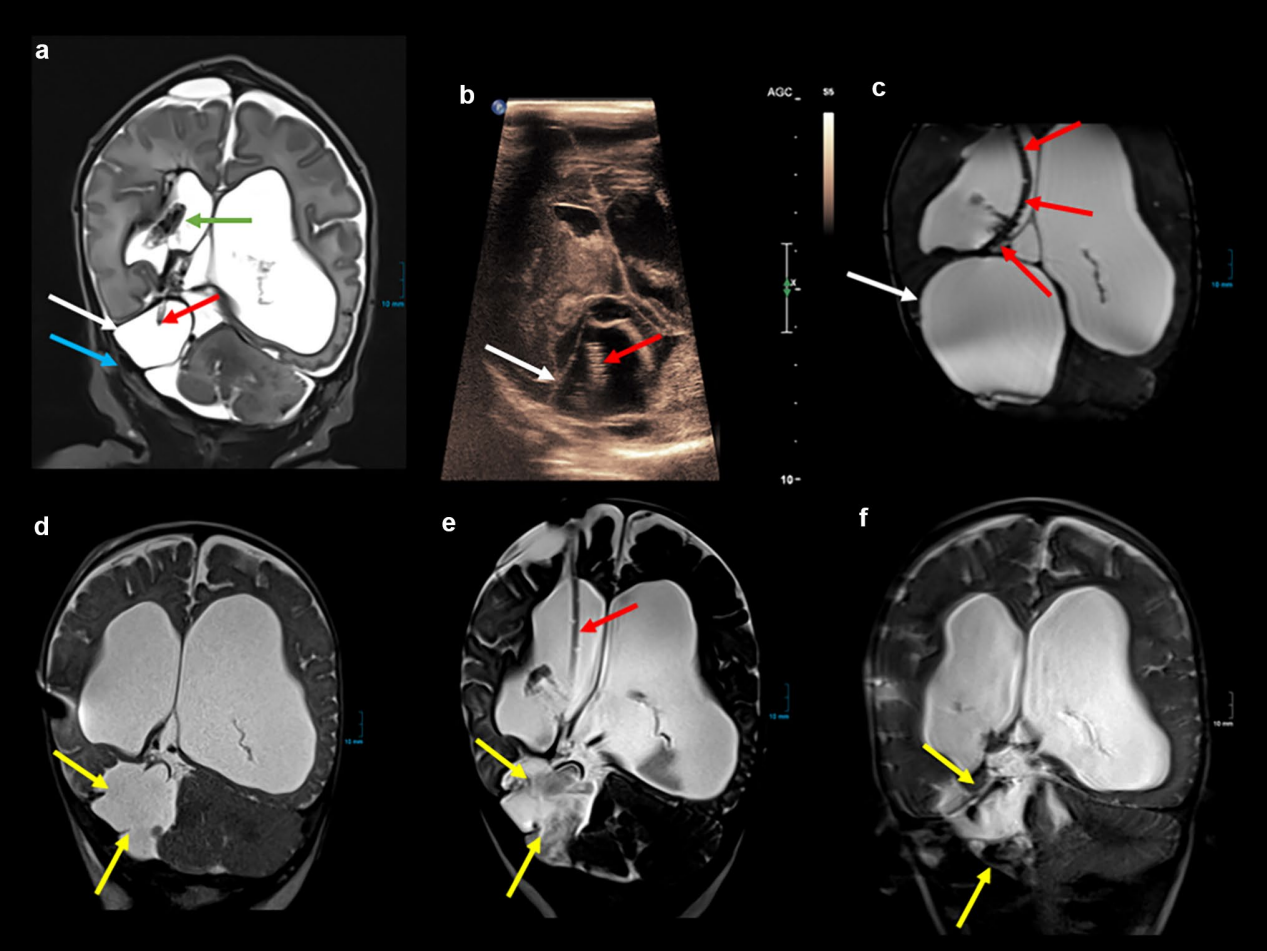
a, b MRI (a: T2 TSE) and transfontanellar ultrasound (b) both in coronal orientations after surgery: ventricular catheter (red arrow) with connection of the cyst (white arrow) with the right lateral ventricle with some bleeding into the ventricle (green arrow); the cyst has developed at the level of the tentorium next to the transverse sinus (blue arrow). c MRI (T2 CISS in coronal multiplanar reconstruction) at 3 months with recurrence of the cyst (white arrow) because the catheter slipped out of the cyst (red arrows). d MRI (T2 HASTE in coronal orientation) after supratentorial minicraniotomy with fenestration of the cyst (supra- and infratentorial, yellow arrows), consecutive reduction in cyst size. e MRI (T2 HASTE in coronal orientation): due to persistent enlargement of the supratentorial ventricular system, a VP shunt with connection to the existing ventricular catheter (red arrow) was established. The fenestration of the cyst (supra- and infratentorial, yellow arrows) is still evident. f Follow-up MRI (T2 HASTE in coronal orientation) at the age of 18 months, with e-vacuo enlargement of the lateral ventricles, the fenestration of the cyst with consecutive flow void artifacts of cerebrospinal fluid (yellow arrows) is still evident
